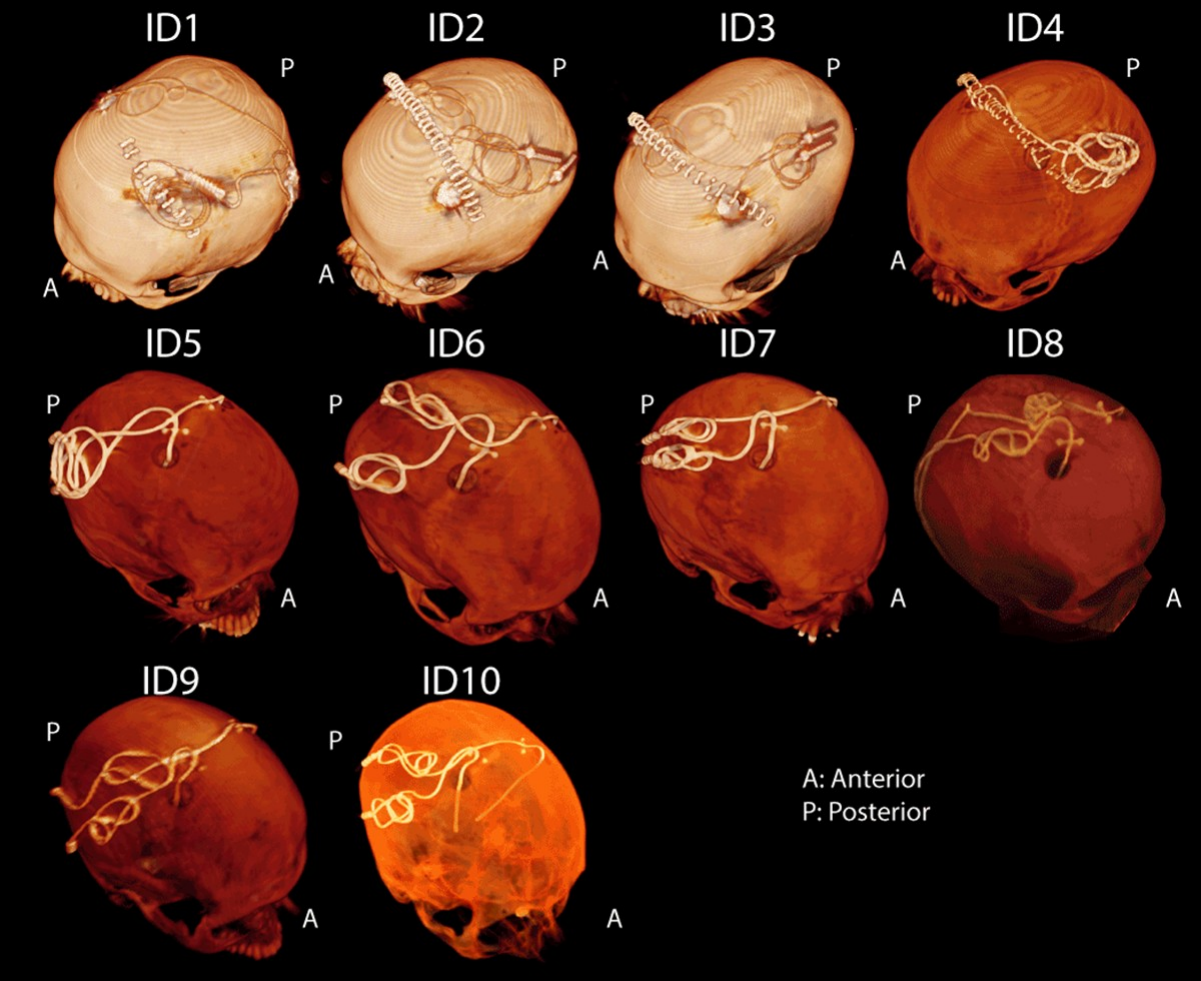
CT images of 10 patients operated for bilateral DBS implantation. For patients 1–4, the IPG was planned to be implanted in the left pectoral pocket. Note that end segments of both leads are routed toward the left side of the head in these patients. In these cases, the left lead was labeled as ipsilateral and right lead was labeled contralateral when representing the temperature results in Figs 6 and 7. For patients 5–10 the IPG was planned to be implanted in the right pectoral region, thus in these patients the right lead was labeled ipsilateral and left lead was labeled as contralateral.
